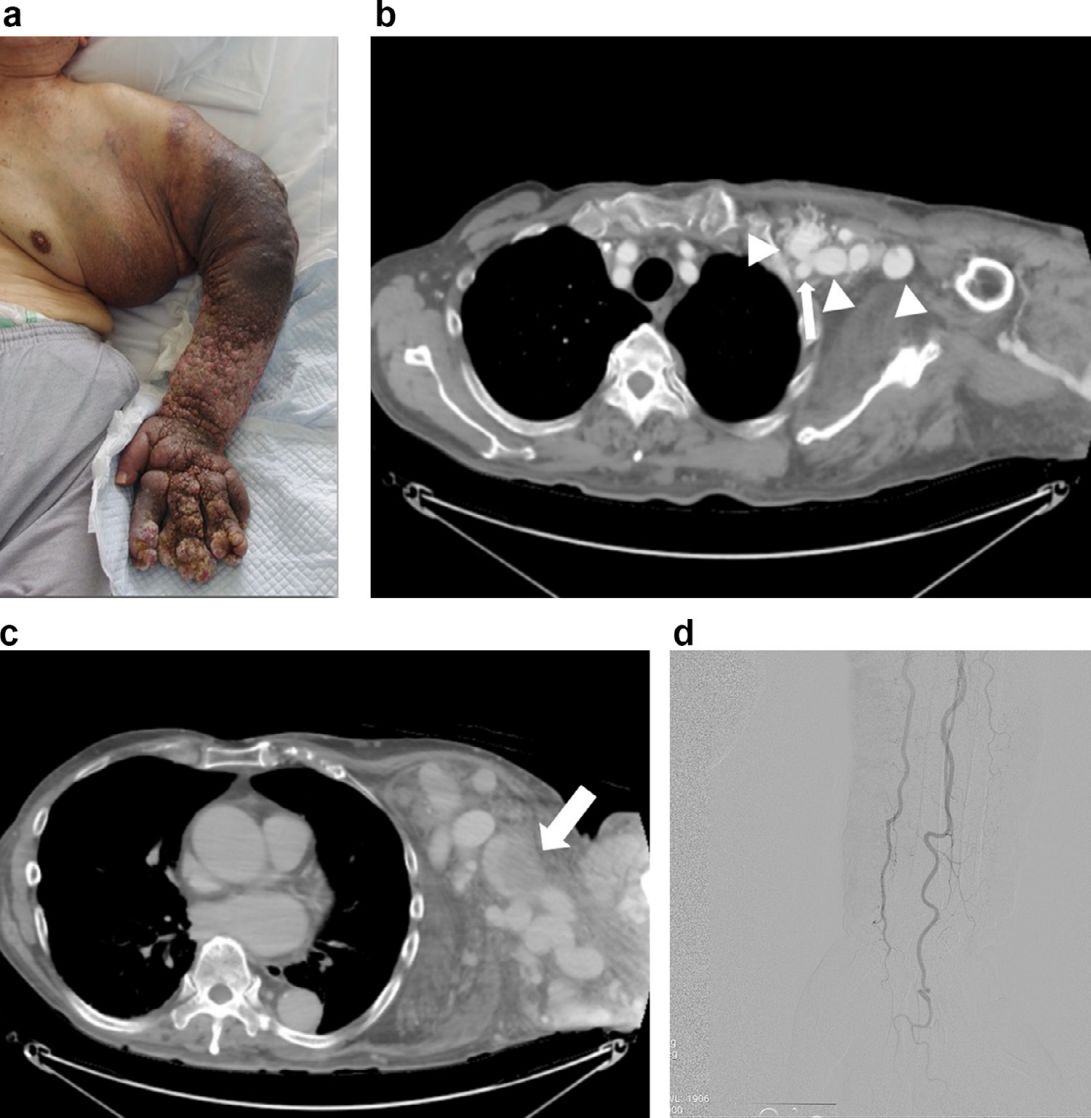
(A) Photograph of the left arm (pretreatment). The left arm has edema, cellulitis, lymphedema, pigmentation of the skin, and infection. (B, C) Preoperative contrast-enhanced CT. (B) Arterial phase. Collateral veins are dilated and enhanced. Arrow: axillary artery. Arrowhead: collateral veins. (C) Equilibrium phase. CT shows thrombus formation in a dilated collateral vein. (D) Digital subtraction angiography. The left arm has no lesion with vessels.
